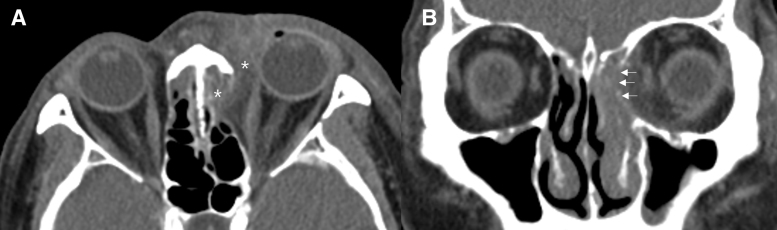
Facial computed tomography images obtained on the day of surgery. (A) Axial view showing a soft tissue mass invading the left ethmoid sinus and medial orbit (*). (B) Coronal view showing bone destruction of the left medial orbital wall (arrows).
